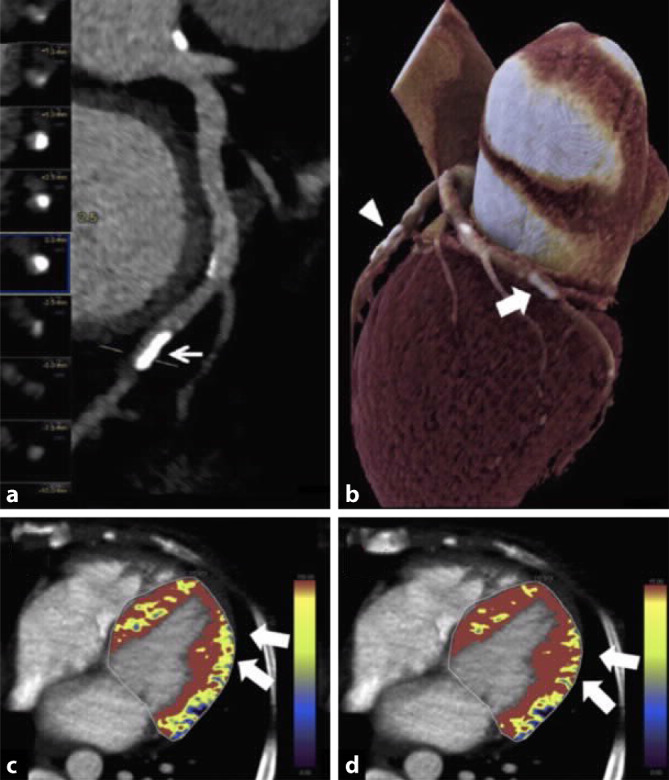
## CT-basierte Messung der fraktionellen Flussreserve
